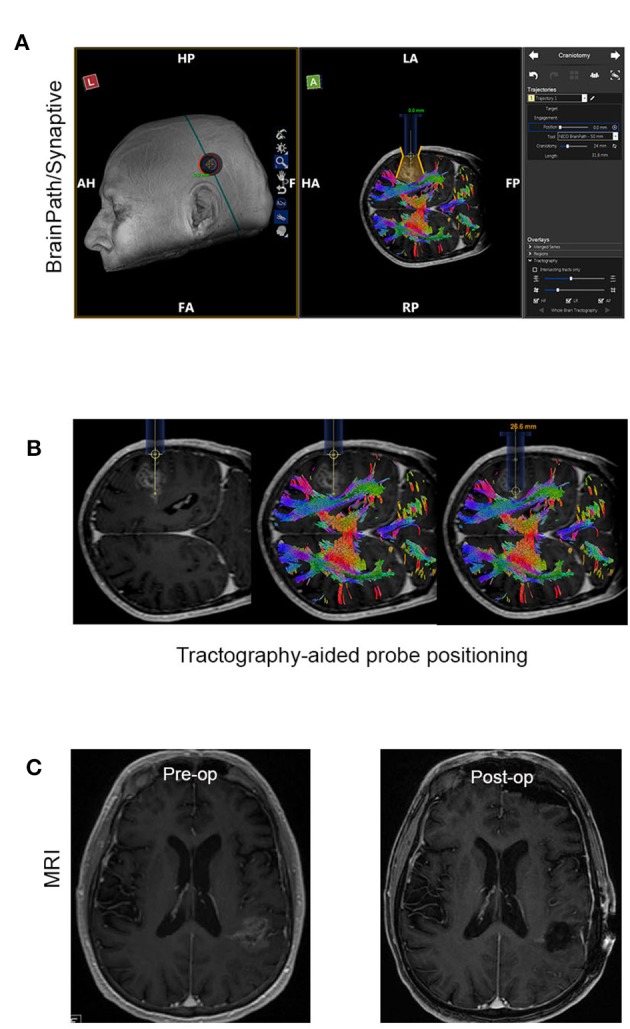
Representative case of a patient that had MIPS resection of the tumor. (A) Use of the planning software (Synaptive BrightMatter) to define a parafascicular trans-sulcal approach to the deep white matter neoplasm. (B) Intraoperative navigation with the target positioned at the deepest enhancing portion of the tumor (left panel). Tractography (shown in the middle panel) suggests that this area disease is within the corticospinal tract and this portion should not be resected. (C) Pre- and post op post gadolinium T1 MRI images with the small “tail” of residual enhancement in the corticospinal tract purposefully left behind.
